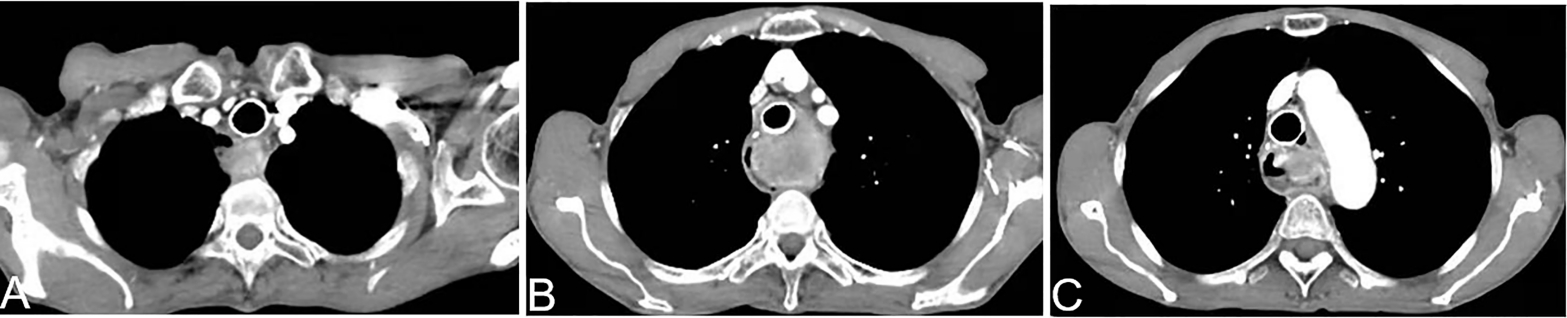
A 69-year-old woman presented with dyspnea of 1-month duration >5 years after esophagectomy. Airway stenting was done 1 week before arterial infusion chemotherapy. The airway lumen was unobstructed. A chest CT scan shows that the soft tissue of the esophagogastric anastomosis was enhanced obviously (A–C).
